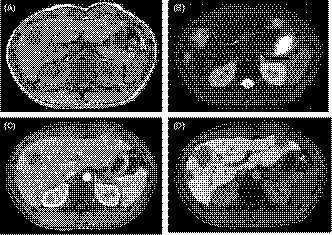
A and B, Magnetic resonance imaging (MRI) showed the tumor with a hypointensity on the T1‐weighted images and a hyperintensity on the T2‐weighted images. C and D, The dynamic MRI study showed the tumor with a heterogeneous enhancement in the early phase and a defect of enhancement in the Kupffer phase
